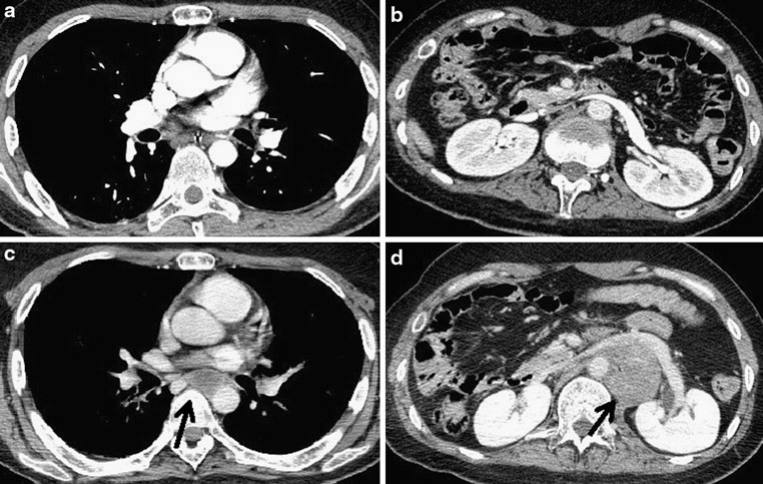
Chest and abdominal CT scans with contrast. a, b Nine months after presentation showing no lesions. c, d Fourteen months after presentation showing tumors in the posterior mediastinum and retroperitoneal space (*arrows*)
